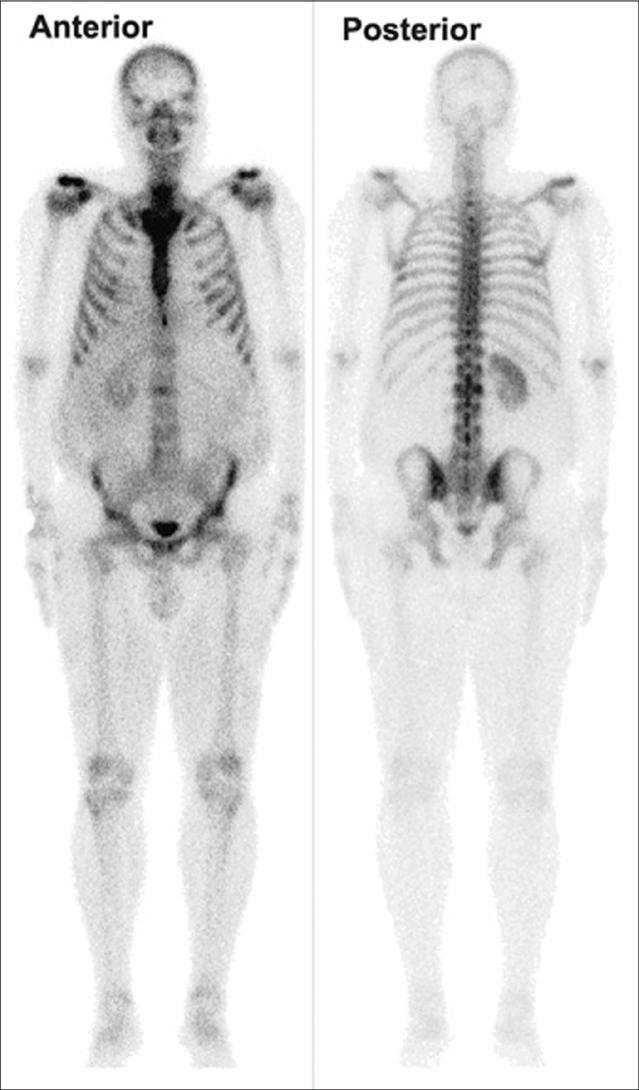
Planar bone scintigraphy performed after I.V. injection of technetium labeled MDP showing very mild increase in the L 2 vertebra prompted a hybrid SPECT-CT imaging of the lumbar spine. Post Left nephrectomy status is also noticed
